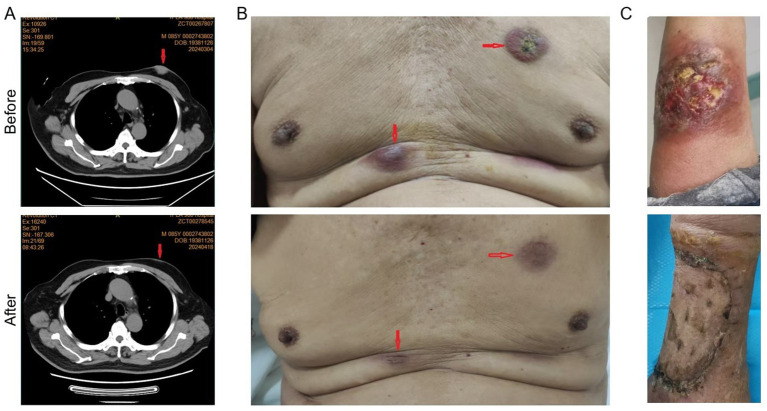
Chest CT scan results and skin recovery of the patient. (A) CT images of skin nodules on the anterior chest; (B) Photos of skin nodules on the anterior chest; (C) Photos of skin ulcer and flap transplantation in the right forearm.
